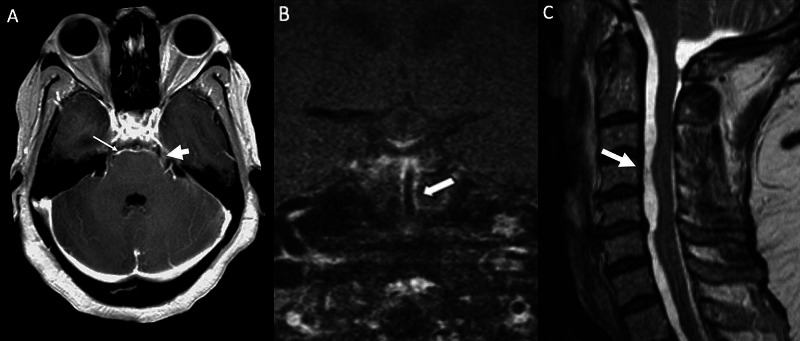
MRI brain and cervical spine Post-contrast axial T1 MR brain (Figure 2A) demonstrates leptomeningeal enhancement along the surface of the pons (arrow) and the cisternal segments of the trigeminal nerves (block arrow). Post-contrast coronal T1 MR (Figure 2B) shows wall enhancement along the basilar artery. Sagittal T2 MR of the spine (Figure 2C) shows loculated CSF collections along the ventral spinal canal in the cervical region associated with cord deformity.
